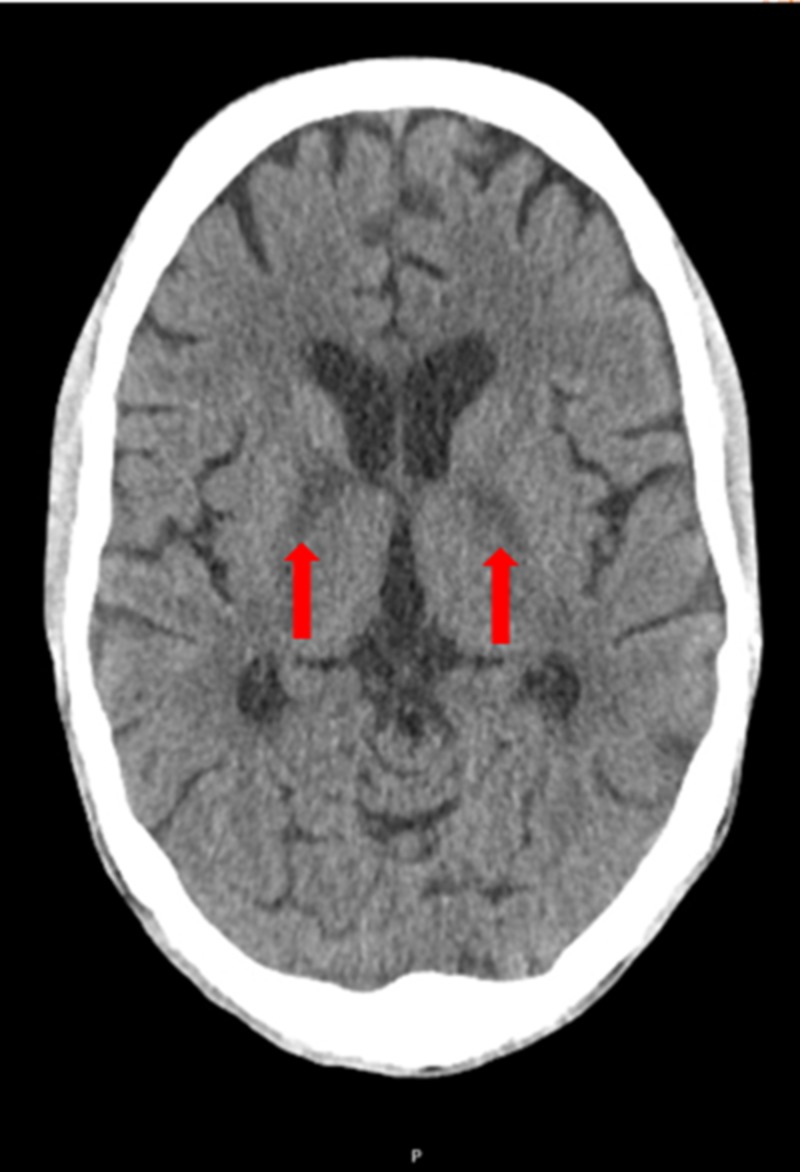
CT of the brain without contrast The image shows new symmetric hyperdensities (red arrows) CT: computed tomography
